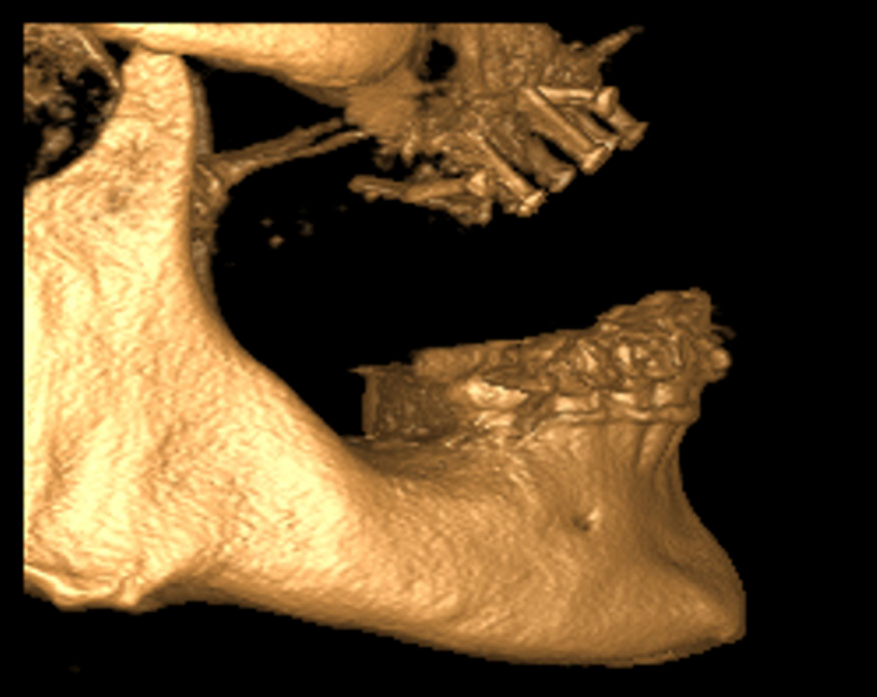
CBCT (3D- rendered; 3DVR, KaVo,) Lateral view before dental implantation and screw-removement
